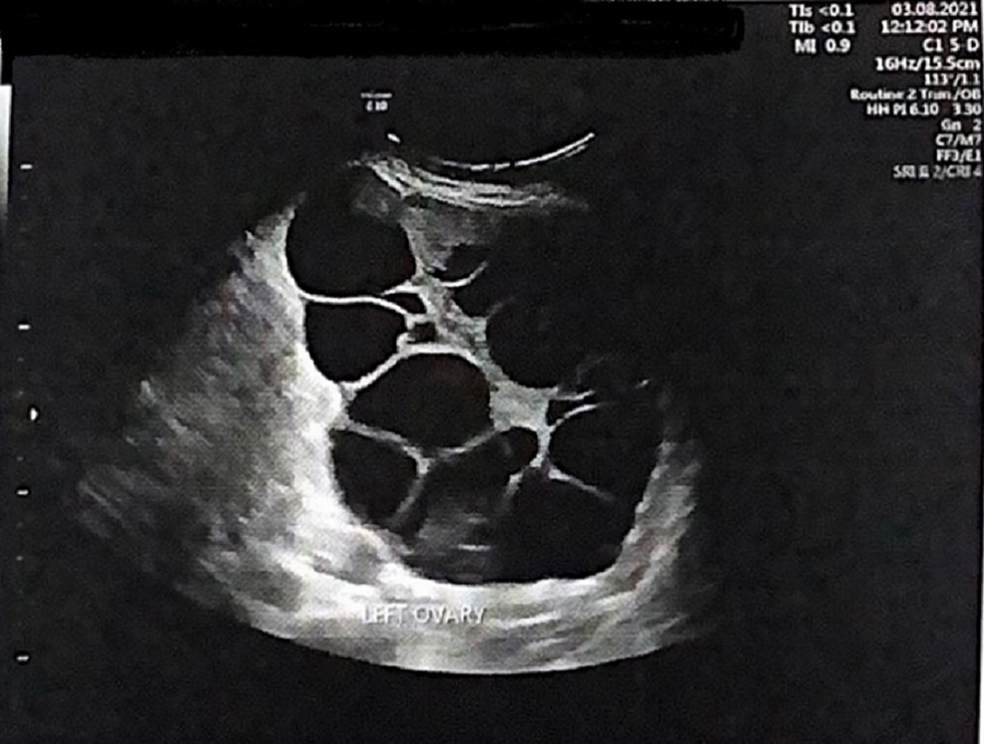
Left ovary Ultrasound scan of abnormally enlarged left ovary, depicting multiple cystic masses.
